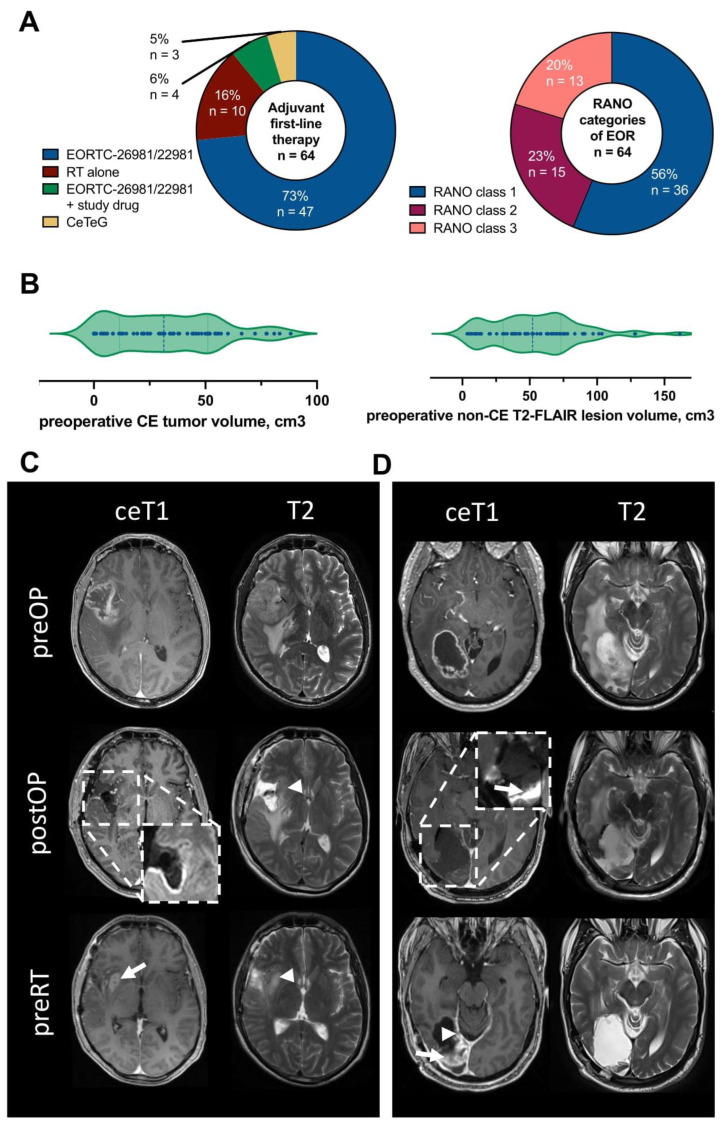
Baseline patient characteristics and volumetric analyses. (A) Distribution of administered first-line adjuvant therapies (left) and stratification of all patients according to the RANO *resect* classes for extent of resection (right) in patients with newly diagnosed glioblastoma CNS WHO grade 4 after undergoing microsurgical tumor resection (*n* = 64). (B) Preoperative CE tumor and non-CE T2-FLAIR lesion volume (in cm^3^) before microsurgical tumor resection (*n* = 64). (C,D) Contrast-enhancing T1- and T2-weighted MRI is shown for two patients displaying volumetric changes in CE and non-CE compartments due to (C) residual non-CE T2-FLAIR lesion progression (arrowheads) and breakdown of the blood–brain barrier leading to new CE tumor (arrow), and (D) postoperative ischemia (arrows in diffusion-weighted imaging inlay) and unspecific changes such as surgical scarring (arrowhead). Abbreviations: CE—contrast-enhancing; ceT1—contrast-enhancing T1-weighted MRI; CeTeG: RT/TMZ → TMZ/CCNU; CNS—central nervous system; EOR—extent of resection; EORTC 26981/22981: RT/TMZ → TMZ; preOP—preoperative MRI; postOP—immediate postoperative MRI; preRT—MRI prior to radio(chemo-)therapy; RANO—Response Assessment in Neuro-Oncology; RT—radiotherapy; non-CE—non-contrast-enhancing; T2—T2-weighted MRI.
